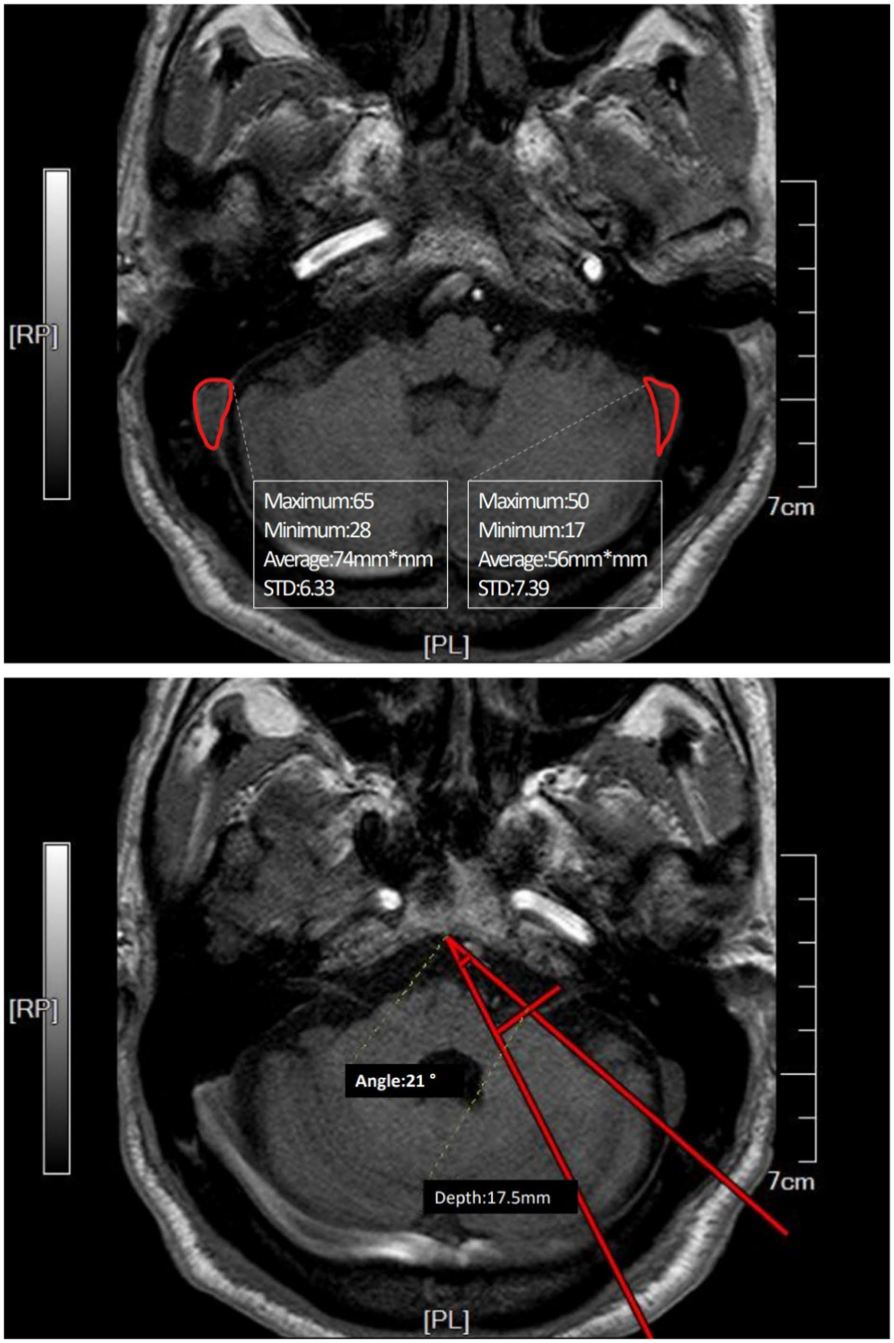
Preoperative MRI image of a case where the affected side and the dominant side are ipsilateral (right side). The area of the sinus was measured in the plane of REZ (root entry zone) where the root of the facial nerve is located. The angle was measured by connecting the midpoint of the REZ plane slope and the sinus border to the responsible blood vessel at its deepest point. The distance from the responsible blood vessel’s deepest point to the perpendicular line from the deepest point of the angle to the petrous bone wall was the depth of the responsible blood vessel.
